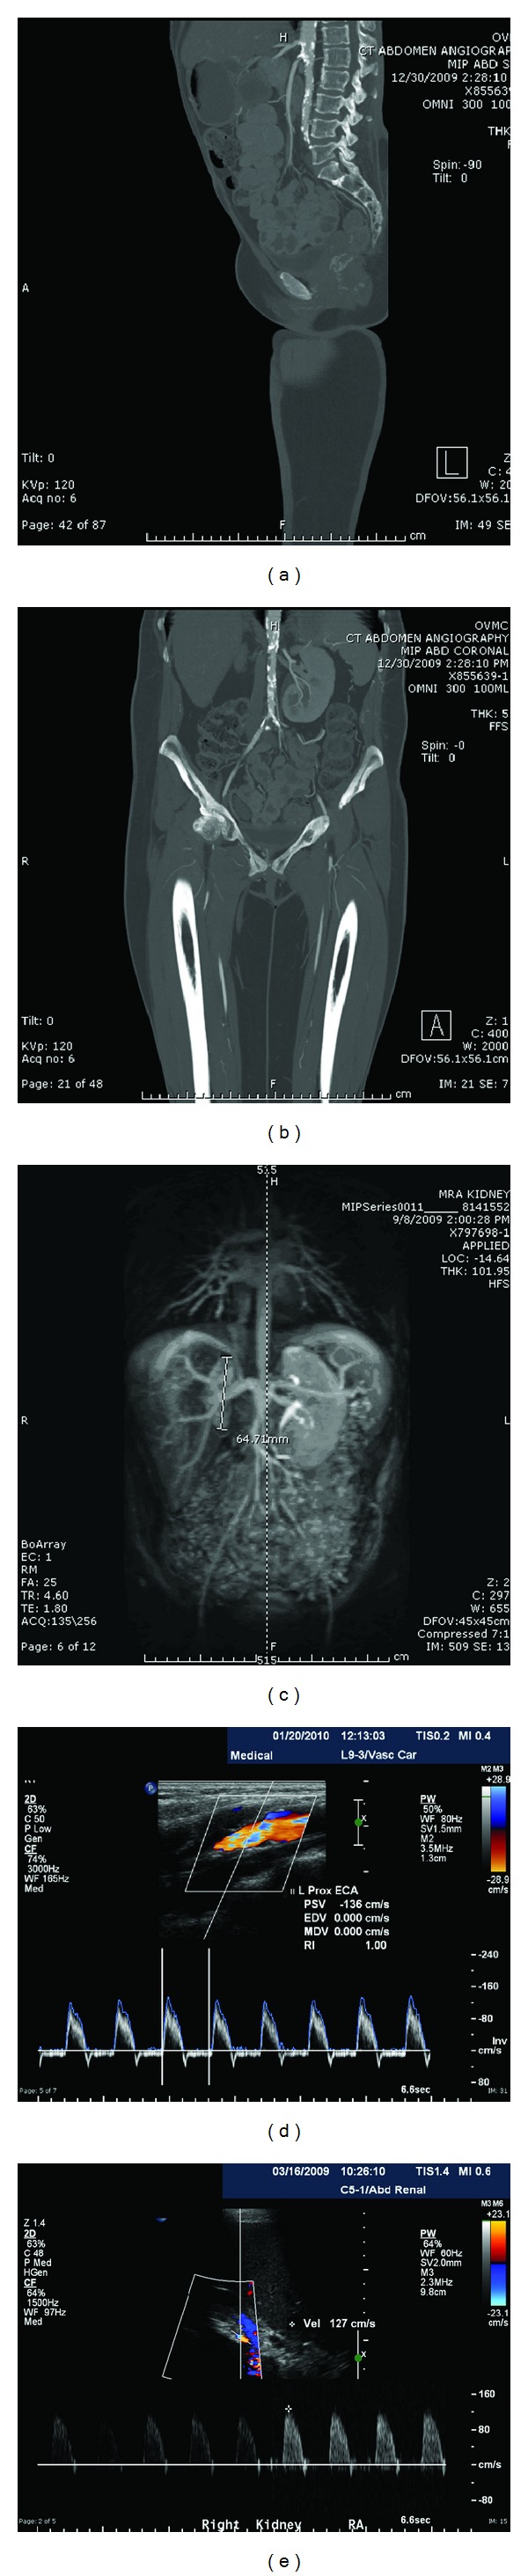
Images of patient J.S. and Takayasu's arteritis (a) Patient J.S. CT angiogram of abdomen and pelvis from 12/31/2009. Sagittal reconstruction showing severe calcifications along the abdominal aorta. (b) Patient J.S. CT angiogram of abdomen and pelvis from 12/31/2009. Coronal reconstructions showing severe abdominal aortic vessel calcification. (c) Patient J.S. MRA of abdomen and pelvis from 09/08/2009. 3D angiogram showing severe abdominal aortic calcifications along length of abdominal aorta, a small right kidney, moderate grade SMA stenosis, low signal in the right renal artery concerning for right renal artery stenosis or right renal artery occlusion, and narrowing of the bilateral iliac and femoral arteries (d) Patient J.S. Doppler flow of left external carotid artery from 01/20/2010. It shows a 50-69% stenosis, as well as notable intimal thickening all along the bilateral carotid arteries, including the left common carotid artery. (e) Renal ultrasound with Doppler from 03/16/2009. It shows an abnormally small right kidney measuring 6.1 cm, right renal artery with high resistance waveform and no diastolic flow suspicious for renal-artery occlusion versus stenosis.
